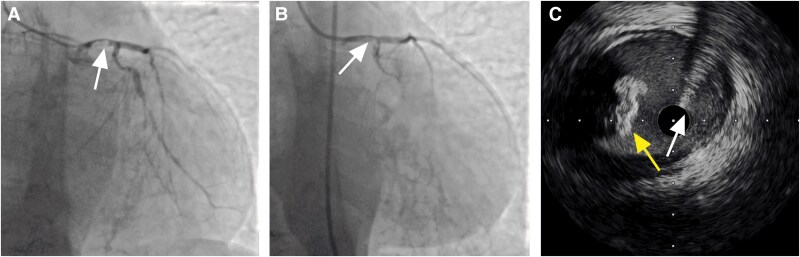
(*A*) Floating thrombus in left main coronary artery on initial invasive coronary angiography (arrow). (*B*) Recurrent thrombus in left main coronary artery/left circumflex artery ostium on repeat invasive coronary angiography (arrow). (*C*) Intravascular ultrasound showing non-obstructive, stable atherosclerotic plaque without evidence of rupture or erosion (yellow arrow) and partial thrombus resolution (white arrow).
